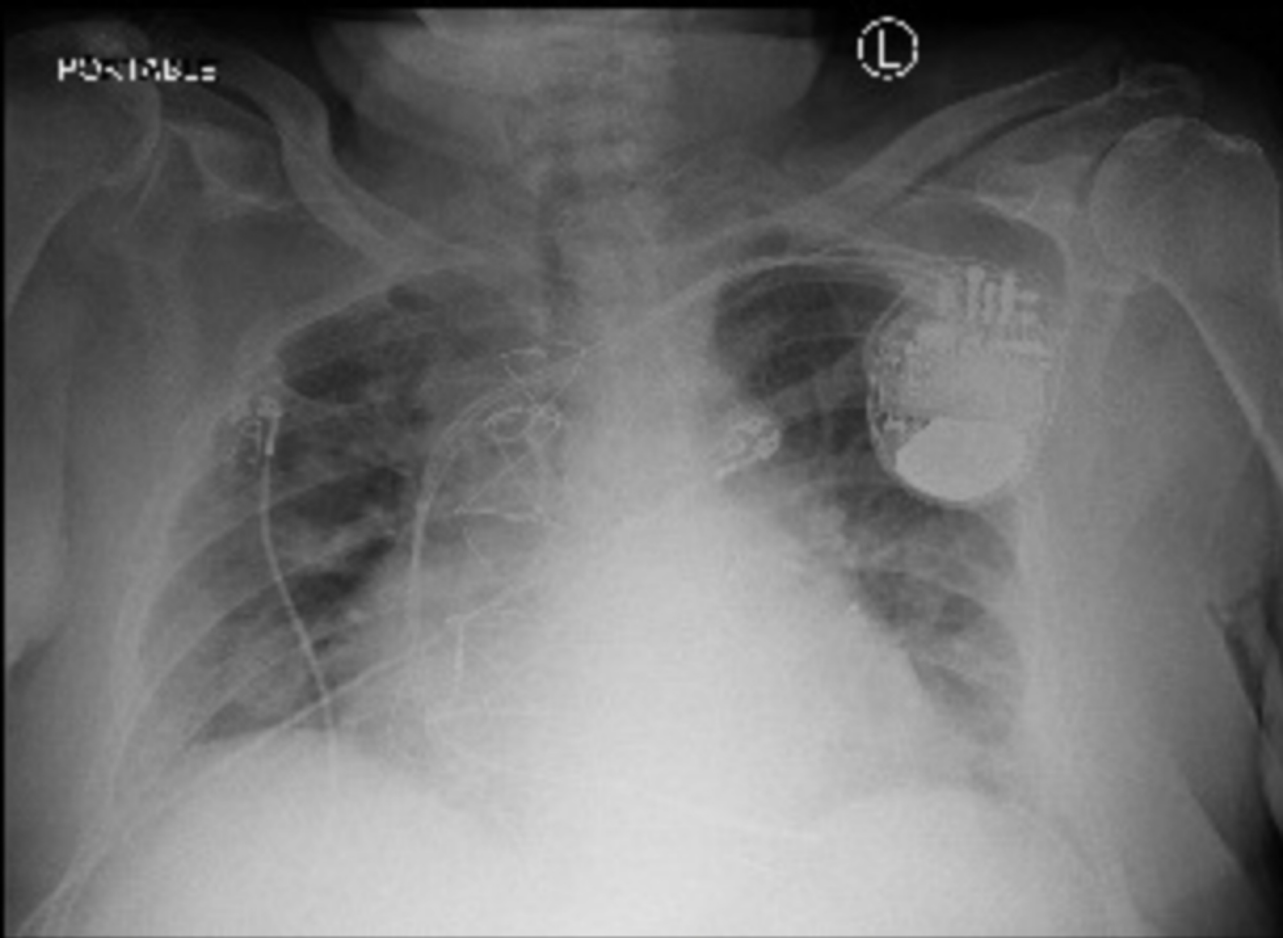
Day 1: portable chest X-ray showing bilateral interstitial infiltrates
